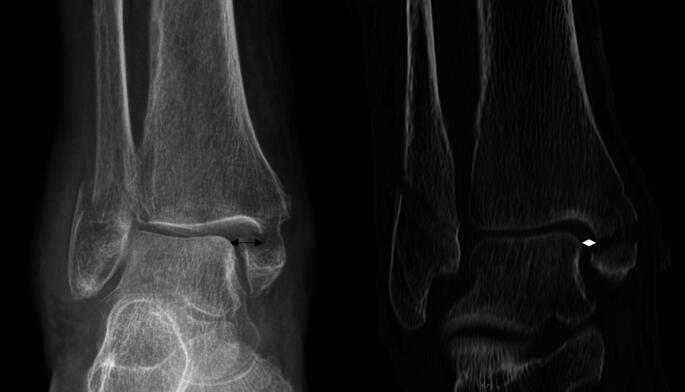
Anterior posterior radiograph of a trimalleolar ankle fracture. The left image shows the ankle in its prereduction state. The black arrow shows the medial clearspace expanded to 8.3 mm. The right image shows the ankle post reduction in computed tomography. The white diamond shows the measured medial clearspace of 4.2 mm
